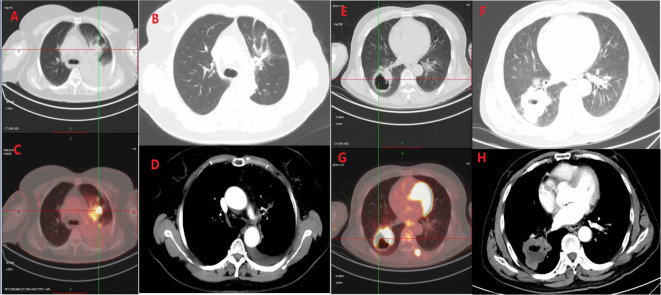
(A) A 63-year-old female patient with stage IVa left upper lobe adenocarcinoma. PET-CT before immunization and chemotherapy showed a SUVmax of 11.0 and an LDH of 196 (A, C). The imaging efficacy evaluation after 6 cycles of treatment showed PR (B, D). (B) A 58-year-old male patient with squamous cell carcinoma of the right lower lobe, PET-CT showed SUVmax of 22.8, LDH of 196 (E, G), and disease progression (F, H) after 4 cycles of treatment.
